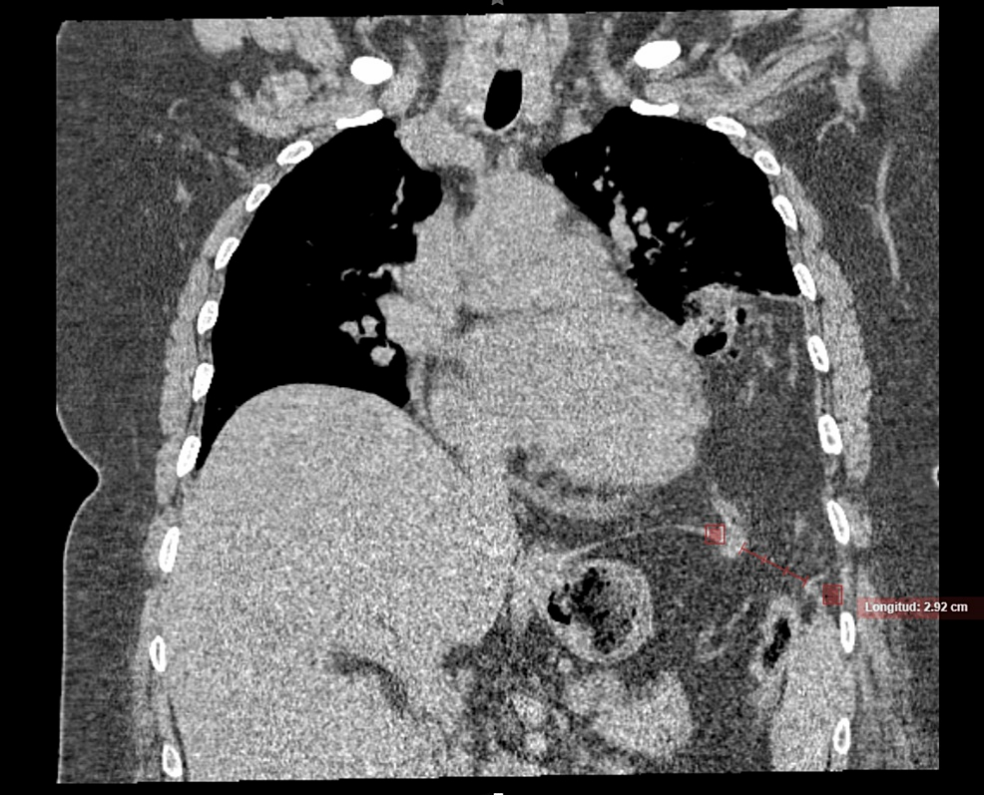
Coronal slice computed tomography (CT) scan: diaphragmatic defect. Diaphragmatic defect with an approximate 2.92 cm diameter.
